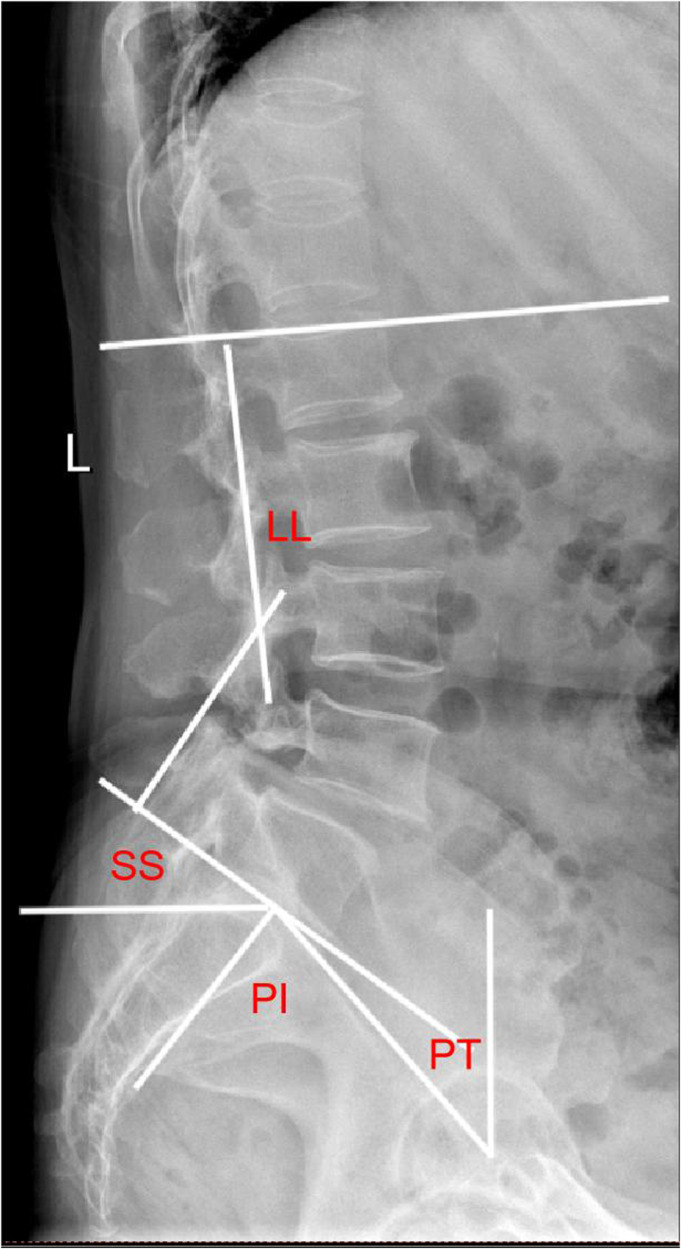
Plain lateral radiograph for measuring radiographic parameters; SL: segmental lordosis; LL: lumbar lordosis; SS: sacral slope; PI: pelvic incidence; PT: pelvic tilt.
